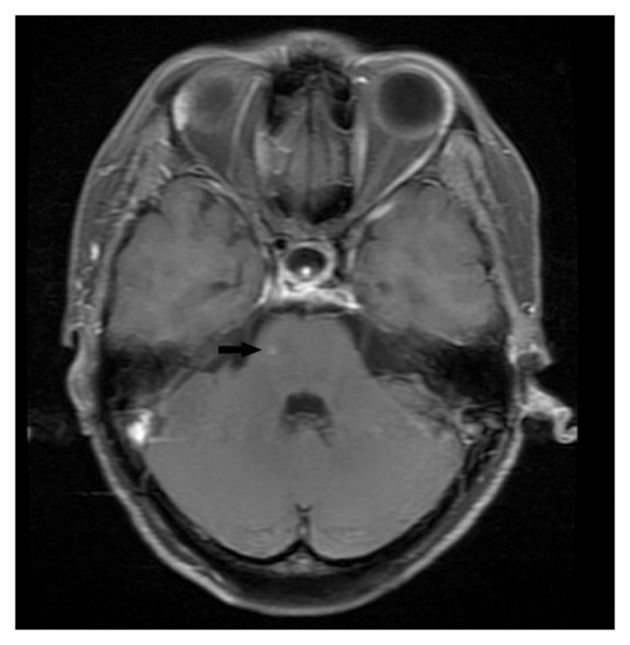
Brain magnetic resonance imaging of a nodule (black arrow) measuring 0.3 cm in the right side of the pons in November 2012.
